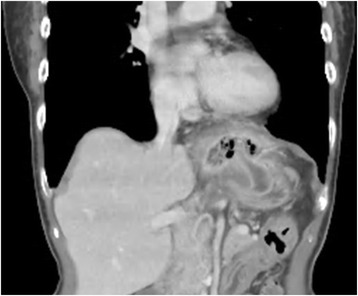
A CT examination shows the extrusion of the mesh into the peri-esophageal region and the esophageal lumen
